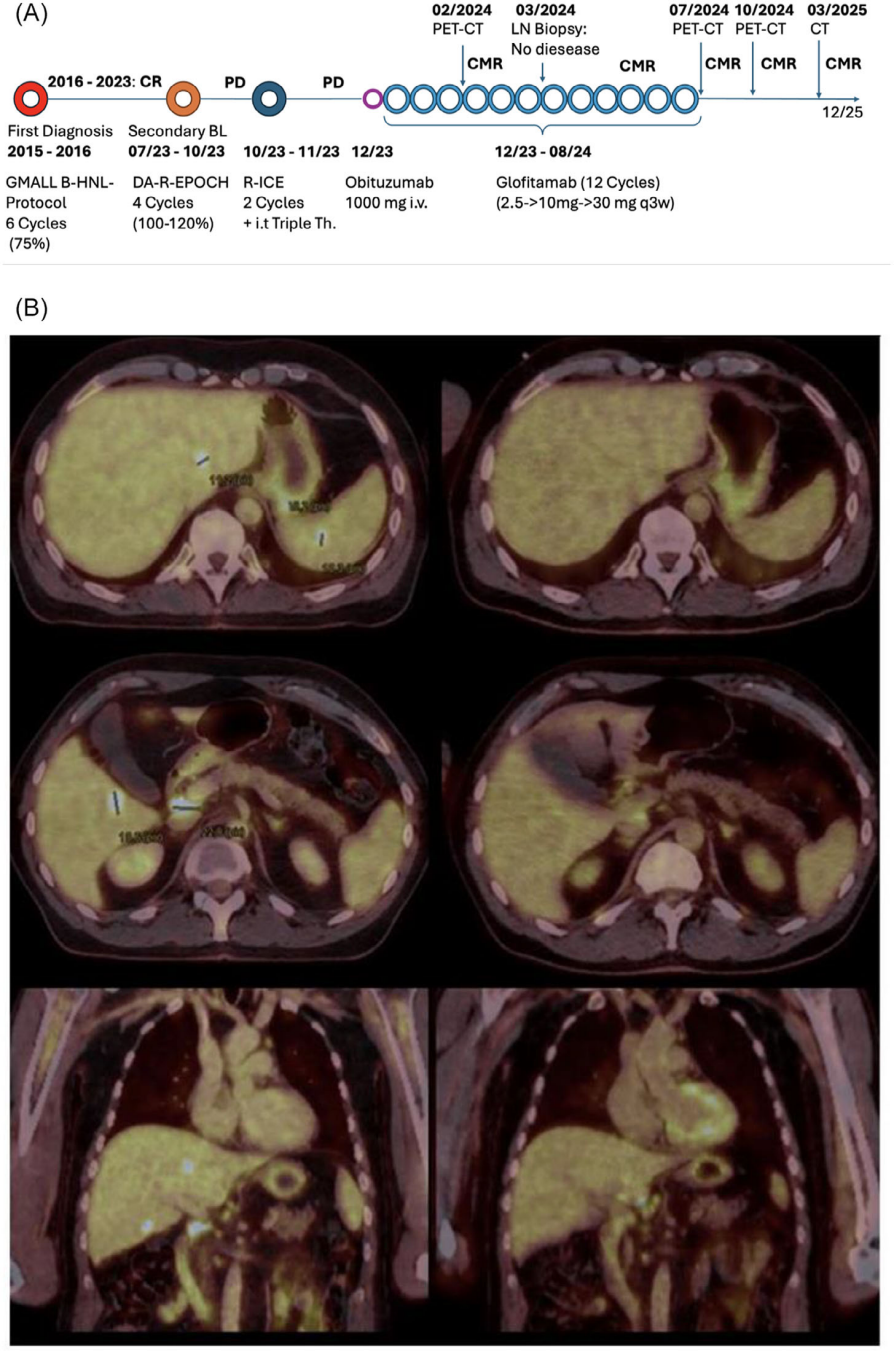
Timeline of treatment and clinical course with positron emission tomography and computed tomography (PET‐CT) comparison. (A) Timeline of treatment and clinical disease course from initial diagnosis to remission. (B) PET‐CT comparison between October 2023 and October 2024 demonstrating complete metabolic response to glofitamab therapy.
